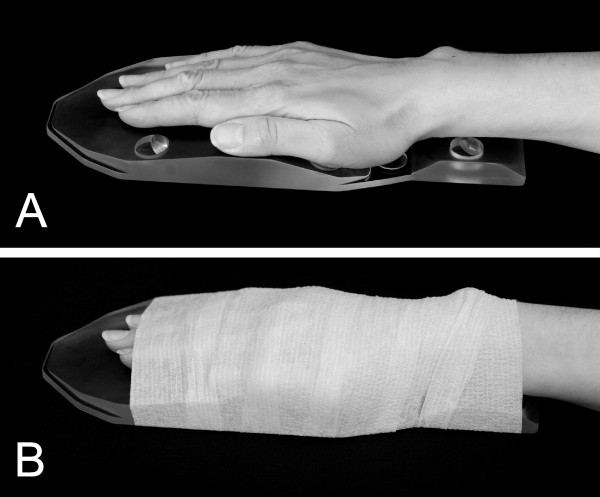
Positioning device used for reproducible alignment of the bones and joints of the hand and wrist during serial magnetic resonance imaging. (A) Hand and wrist are shown positioned on the acrylic M-frame™ with the fingers and thumb adducted and in plane with each other. (B) The hand and wrist are secured to the frame with self-adhesive, latex-free, elastic bandage (images courtesy of Spire Sciences, Inc).
